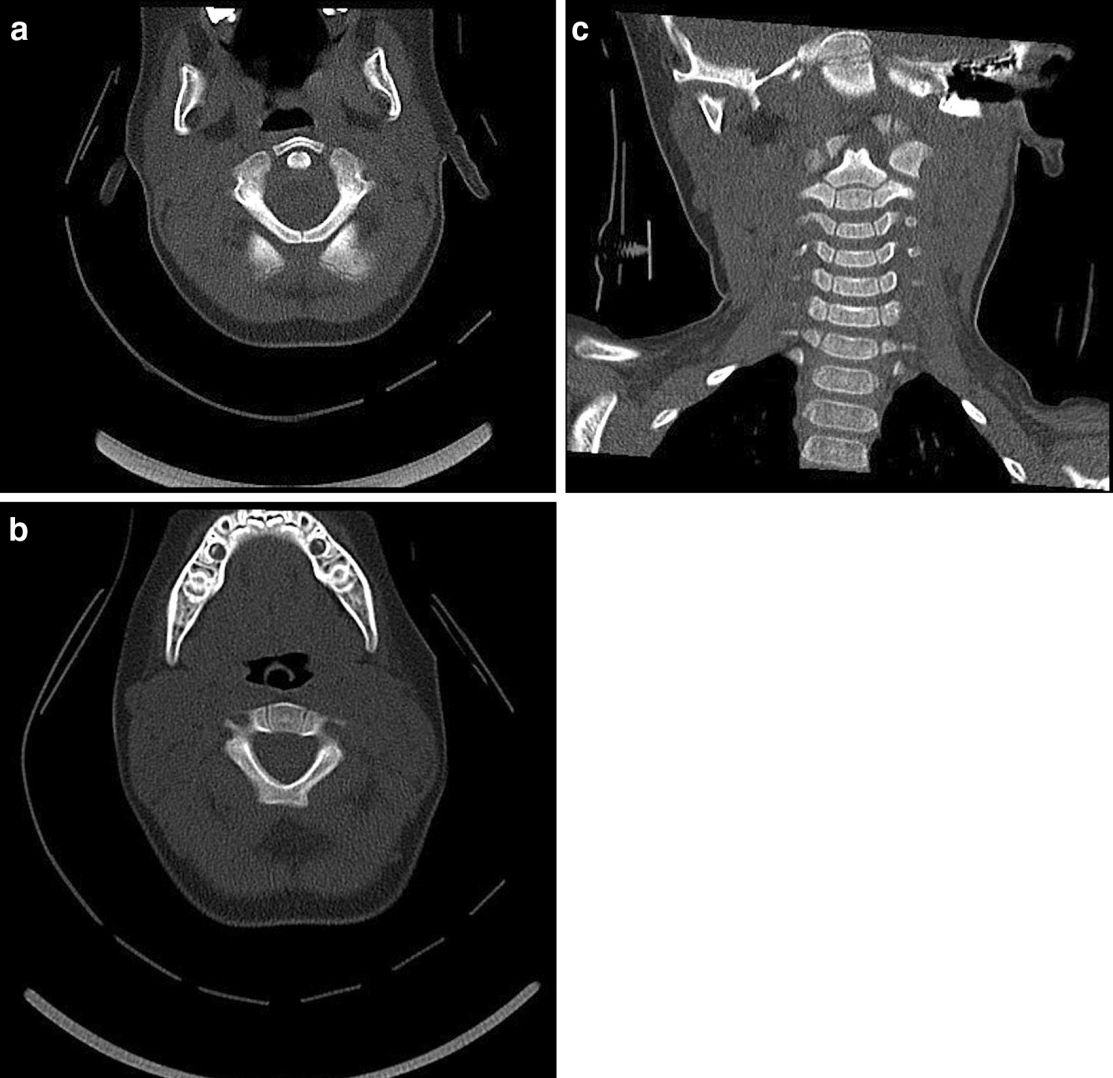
CT images demonstrating synchondroses of: a C1 on axial view; b C2 on axial view; and c the subaxial spine on coronal view
